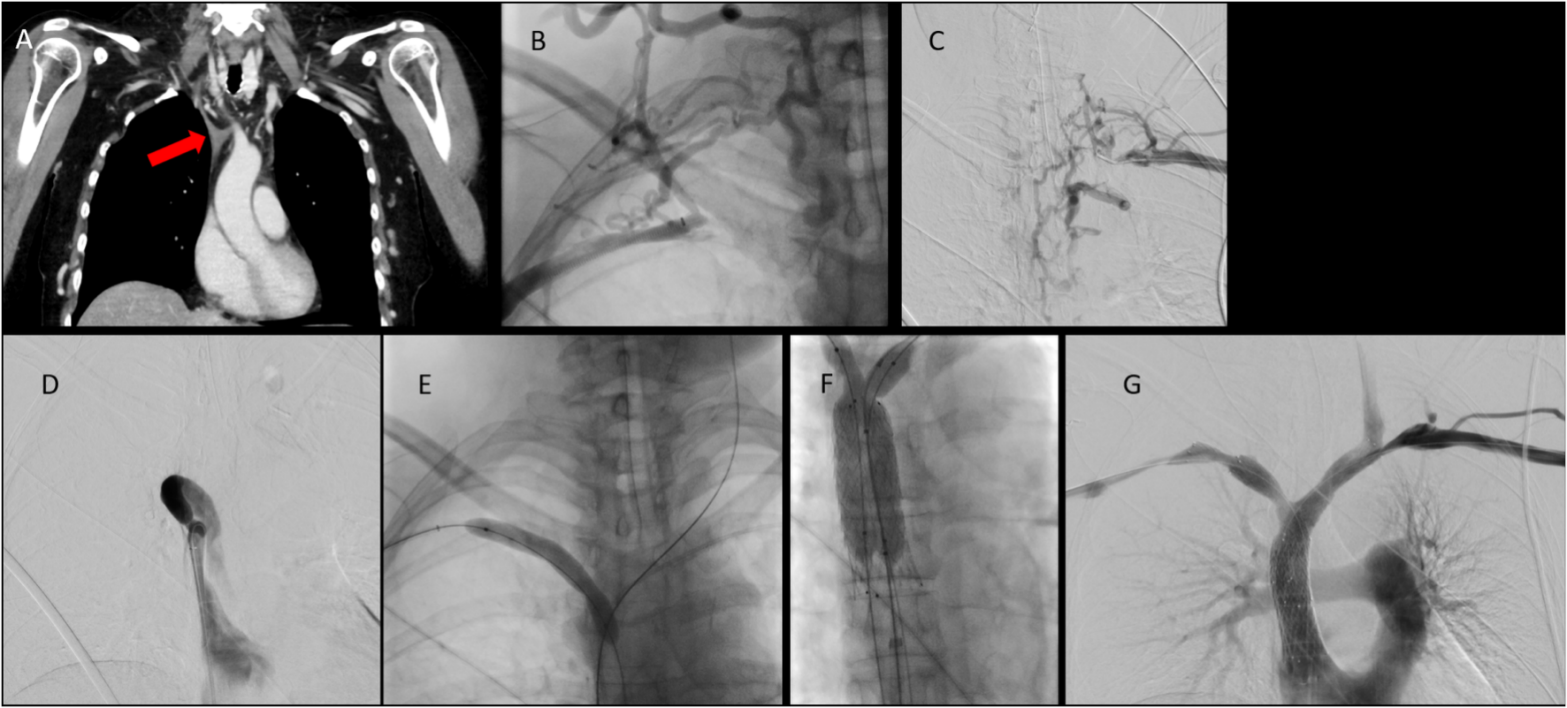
43-year-old female patient with short bowel syndrome and multiple port-a-cath implantations over the last years presenting with chronic occlusion of the brachiocephalic vein on both sides as well as the superior vena cava, resulting in a severe post-thrombotic superior vena cava syndrome; A: Coronal CT image showing the occlusion of the SVC and both brachiocephalic veins (red arrow in A). B and C: Right and left brachial venous access with phlebography confirming the occlusions from the right (B) and left side (C). D: Occlusion of the SVC from the caudal (femoral) access. E: Wire passage from anterograde and retrograde. Two flossing wires in place. Ballooning of the right brachiocephalic vein. F: Y-shaped reconstruction the occlusion of SVC with one 20 mm Venovo Stent (BD Bard) and two 12 mm Venovo Stents in each brachiocephalic vein. G: Final phlebography showing the SVC reconstruction
